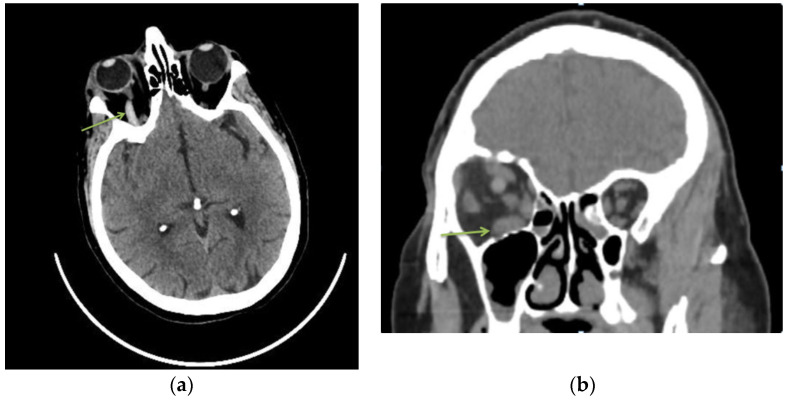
A 77-year-old woman, with hypertensive pathology, presented to the emergency department complaining of eye ptosis, chemosis and conjunctival congestion. The patient was known to have high myopia (both eyes) and amblyopia (LE). The ophthalmological examination revealed best-corrected visual acuity (BCVA) on the right eye 0.5 and 0.06 for left eye. Intraocular pressure (IOP) was 25 mmHg in RE and 14 mmHg in LE. The anterior segment of the left eye was normal. In the RE we noticed diffuse conjunctival congestion, chemosis and eyelid ptosis. Ocular movements were normal in both eyes. Pupillary light reflex was bilaterally intact. Hertel’s exophthalmometry revealed a proptosis of 20 mm in the right eye vs. 17 mm in the left eye. Non-contrast computed tomography (CT) of the brain and orbits revealed a hyperreflectivity at the level of the right ophthalmic vein (a) and inferior rectus muscle hypertrophy (b). Routine biochemical examination showed an increase of inflammatory markers (C-reactive protein, fibrinogen) and a high D-dimers value. Blood culture and a bacteriological conjunctival exam were negative. Additionally, other secondary causes were excluded (tumors, autoimmune diseases, infections). (a) Axial sections of non-contrast head CT scan—hyperreflectivity of the right ophthalmic vein. (b) Non-contrast head CT coronal scan—inferior rectus muscle hypertrophy.
